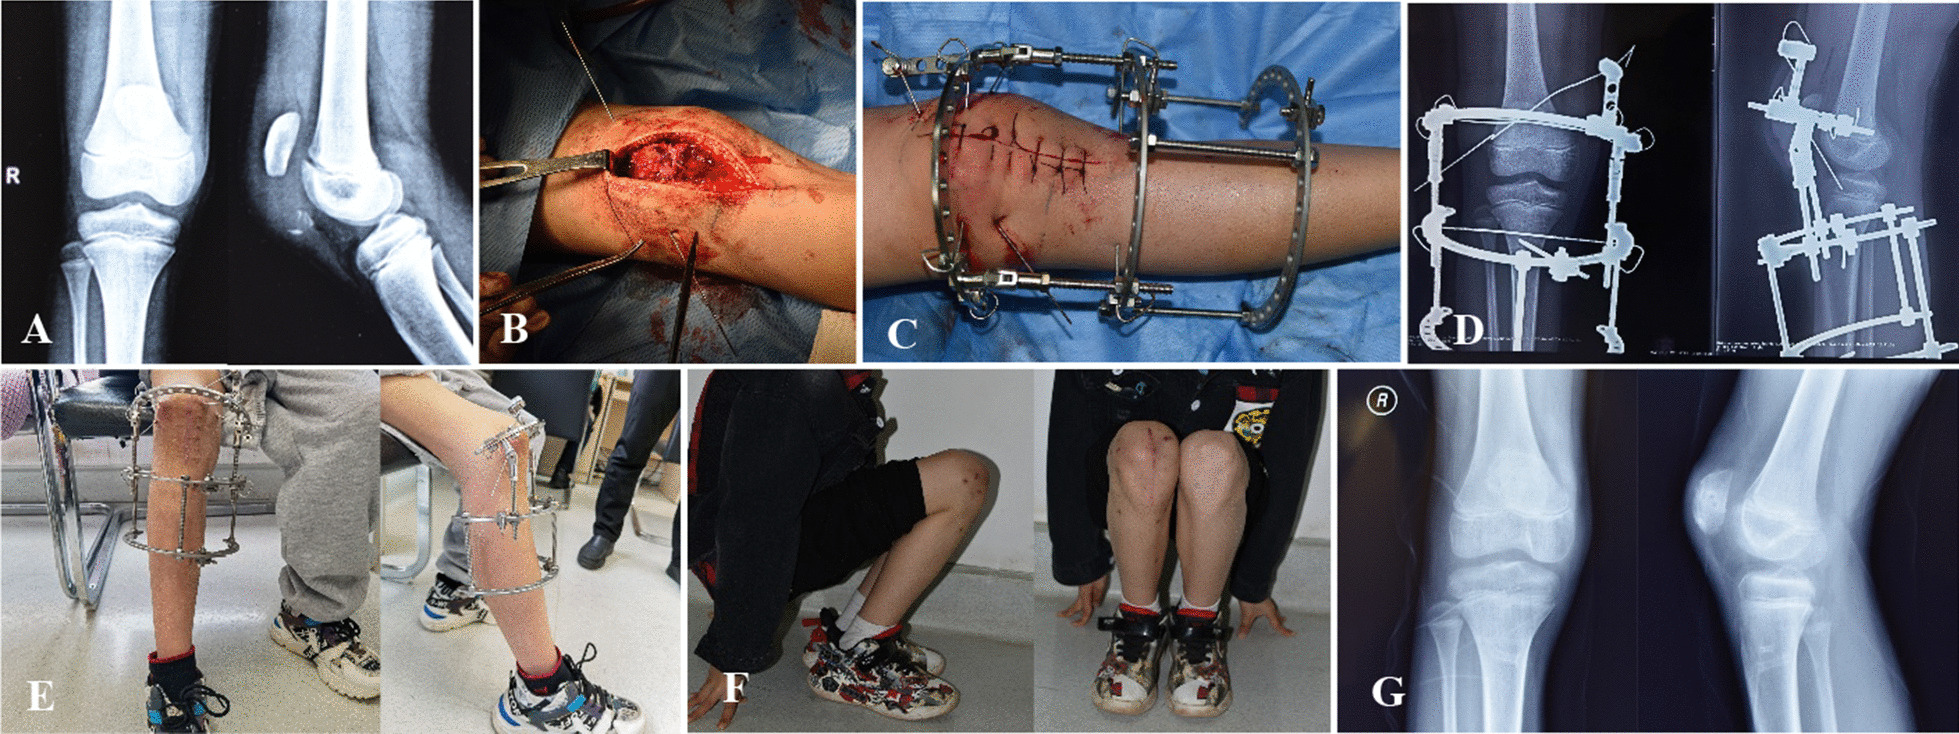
A 18-year-old male patient with right IPFP due to a fall. A Anteroposterior and lateral X-rays of the right knee before surgery. B After avulsion fracture reduction, the fracture block was immobilized using Kirschner wires with a blocking head, and 1-gauge absorbable sutures were used for suture immobilization. C Postoperative tension-free external immobilization device. D Anteroposterior and lateral X-rays of the right knee after surgery. E Postoperative frame-carrying conditions. F The knee function was good after removal of the external immobilization frame. G Anteroposterior and lateral X-rays of the right knee after removal of the external immobilization frame
